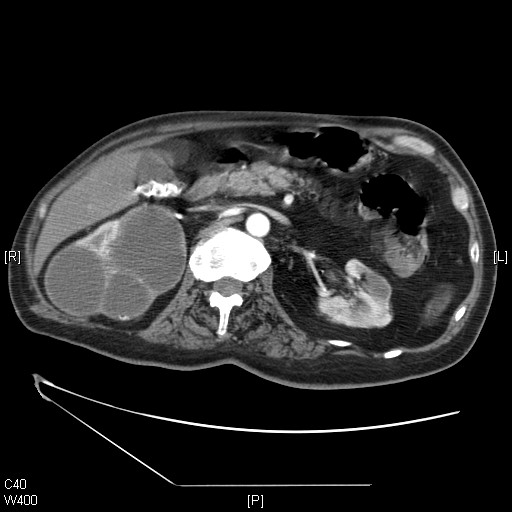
Axial CT scan of the abdomen, performed on 7 July 2003. Several cysts are shown in the right kidney, the largest measuring 6 cm in diameter. The outline of the right kidney was intact. The perirenal fat was seen without any discontinuity. The gall bladder contained several stones.
